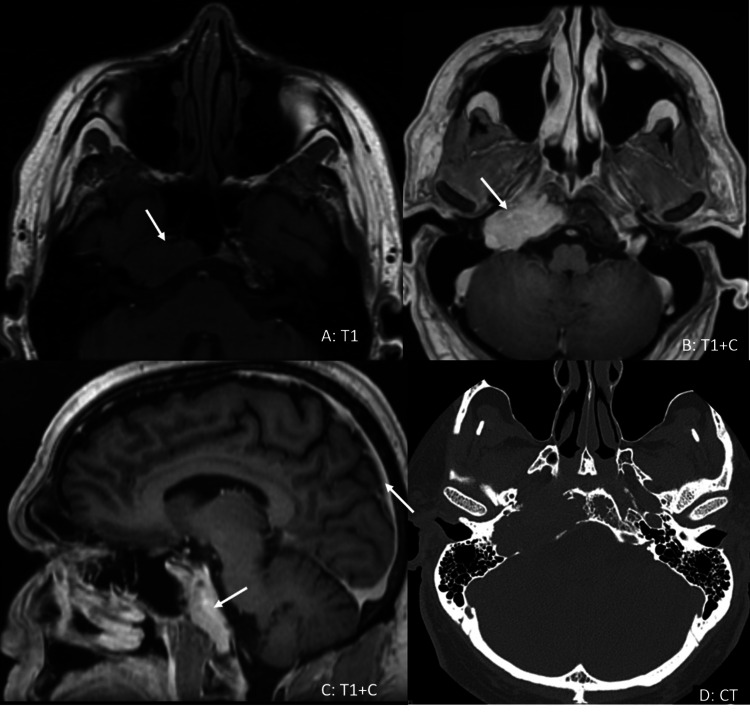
MRI findings Axial pre-contrast T1 (A) and post-contrast T1-weighted images (B) demonstrate a large expansile mass (arrow) involving the right side of the clivus, petrous apex and right jugular foramen. Sagittal post-contrast T1-weighted images (C) demonstrate an enhancing mass replacing the entire right side of the clivus. Axial bone window CT images (D) show an expansile lytic lesion (arrows) on the right side of the clivus and petrous apex, extending to the jugular foramen. The mass involves the expected location of Dorello’s canal.
